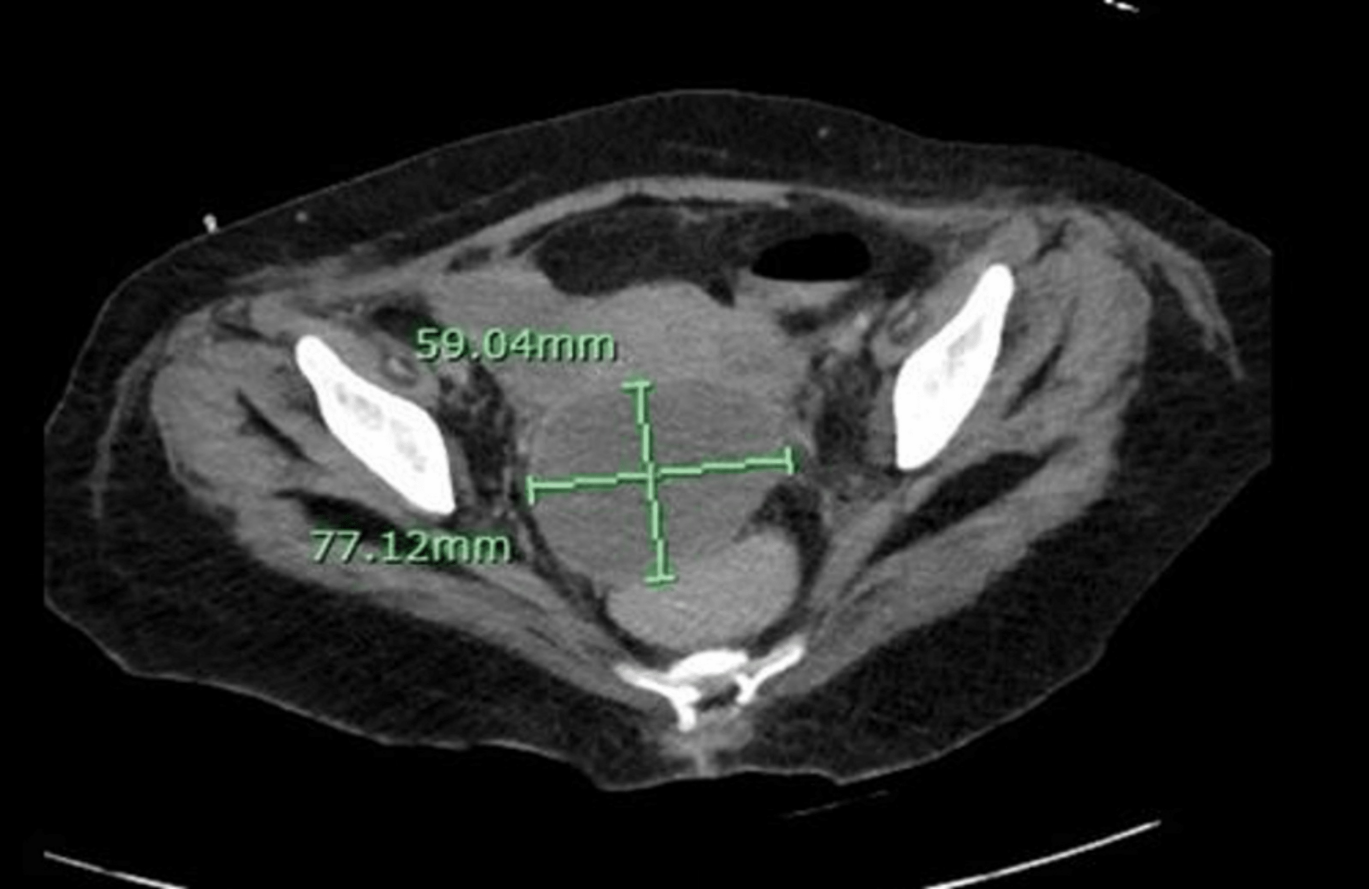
CT scan showing a paracoccygeal abscess
